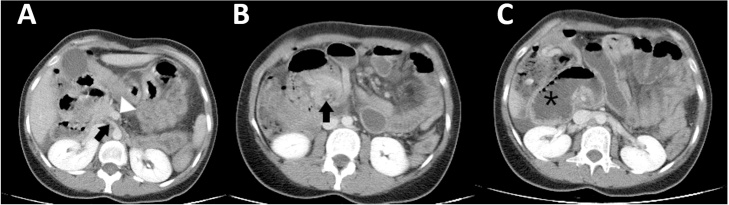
Axial contrast-enhanced CT images of the upper abdomen. (A) Superior mesenteric artery (black arrow) is abnormally located to the right of the superior mesenteric vein (white arrowhead). (B) Abnormally positioned small bowel around the SMA (black arrow) with a characteristic swirling pattern, consistent with midgut volvulus. (C) Small bowel dilatation (asterisk) secondary to small bowel obstruction due to midgut volvulus.
